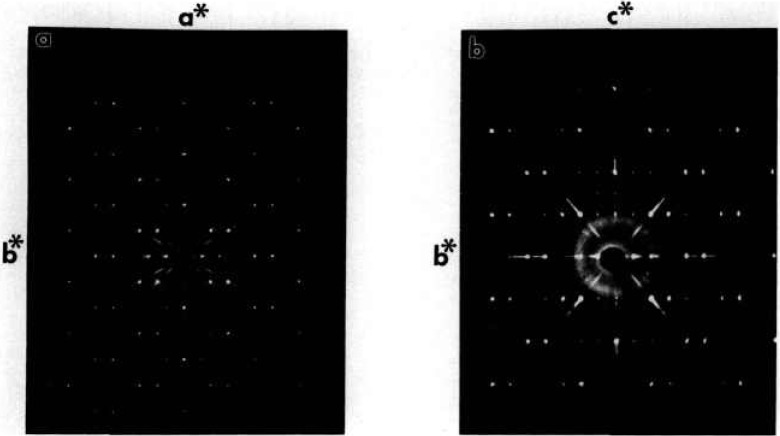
X-ray precession photographs of Ca_4_Bi_6_O_13_ (Mo radiation), (a) *(hk0*), (b) (*0ld*).
